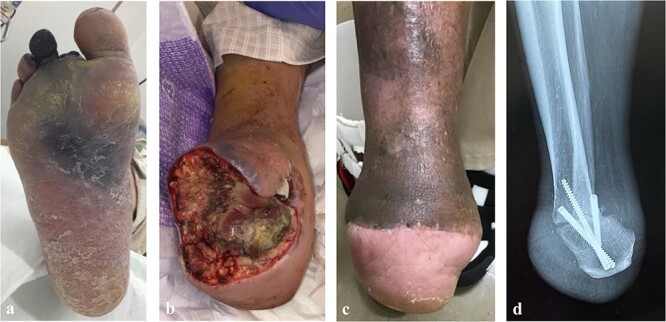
The postoperative clinical picture of case 1; (a) the infection spread to the third, fourth digits, and plantar side; (b) the clinical picture of after transection at the Chopart joint; (c) the clinical picture after Pirogoff amputation; (d) lateral radiography after Pirogoff amputation.
